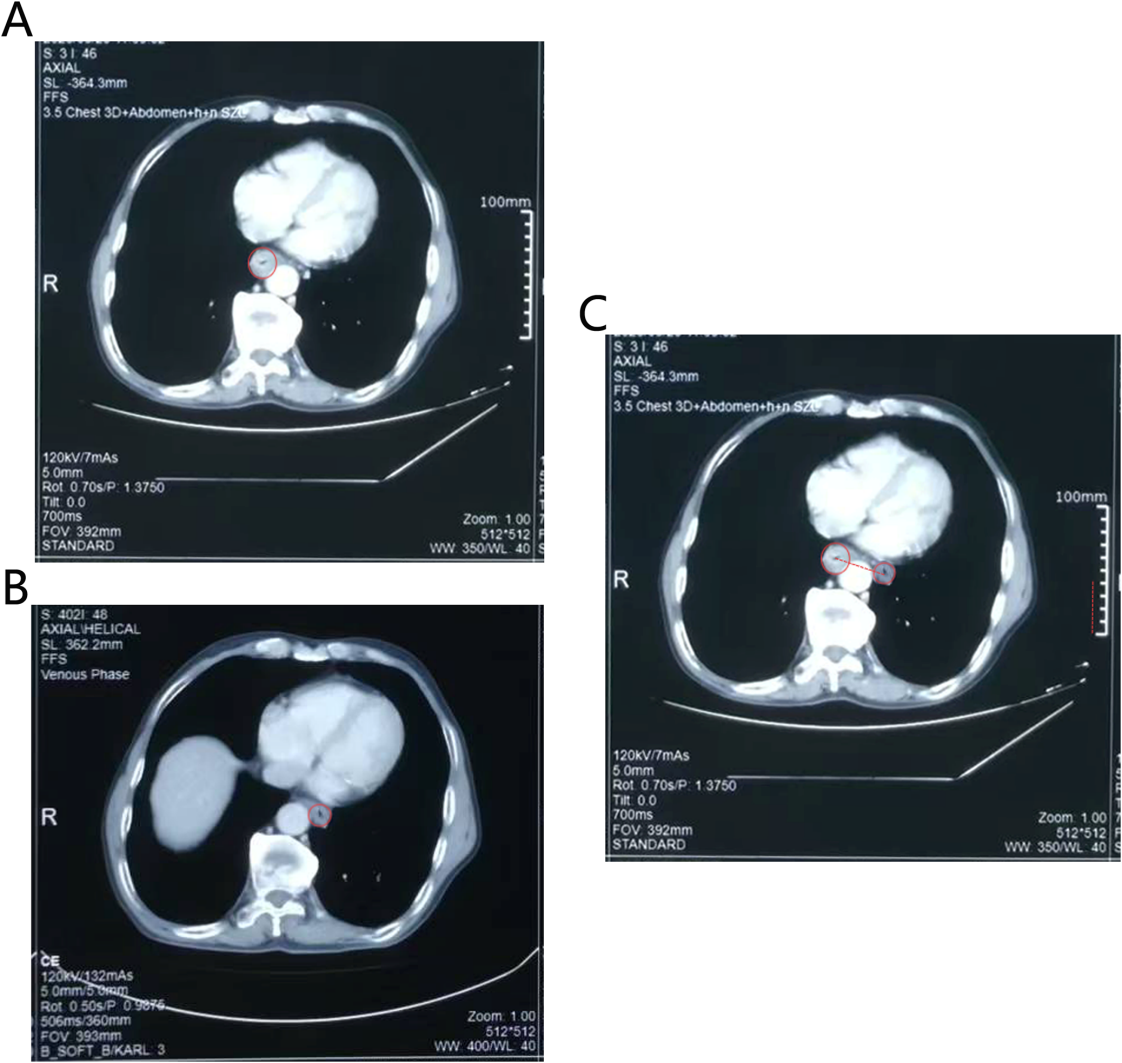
The CT images showed that esophagus had a dramatic radial escape after treatment. (A) The position of the esophagus before treatment. (B) The position of the esophagus after treatment. (C) The changes in the position of the esophagus before and after treatment. The red dotted line represented the distance that the esophagus moved. Technical details was in the CT images (e.g., slice thickness: 5mm).
